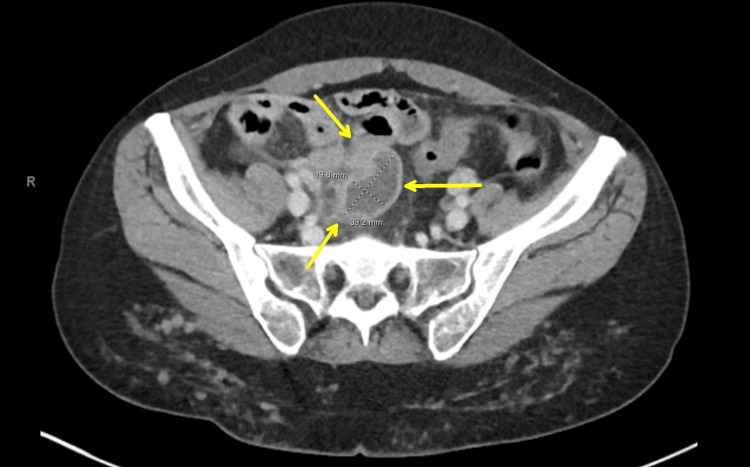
Computed tomography scan of the abdomen and pelvis (CTAP) showing a 3.9 x 1.98 x 3.7 cm rim-enhancing complex fluid collection in the right adnexa (yellow arrows).
